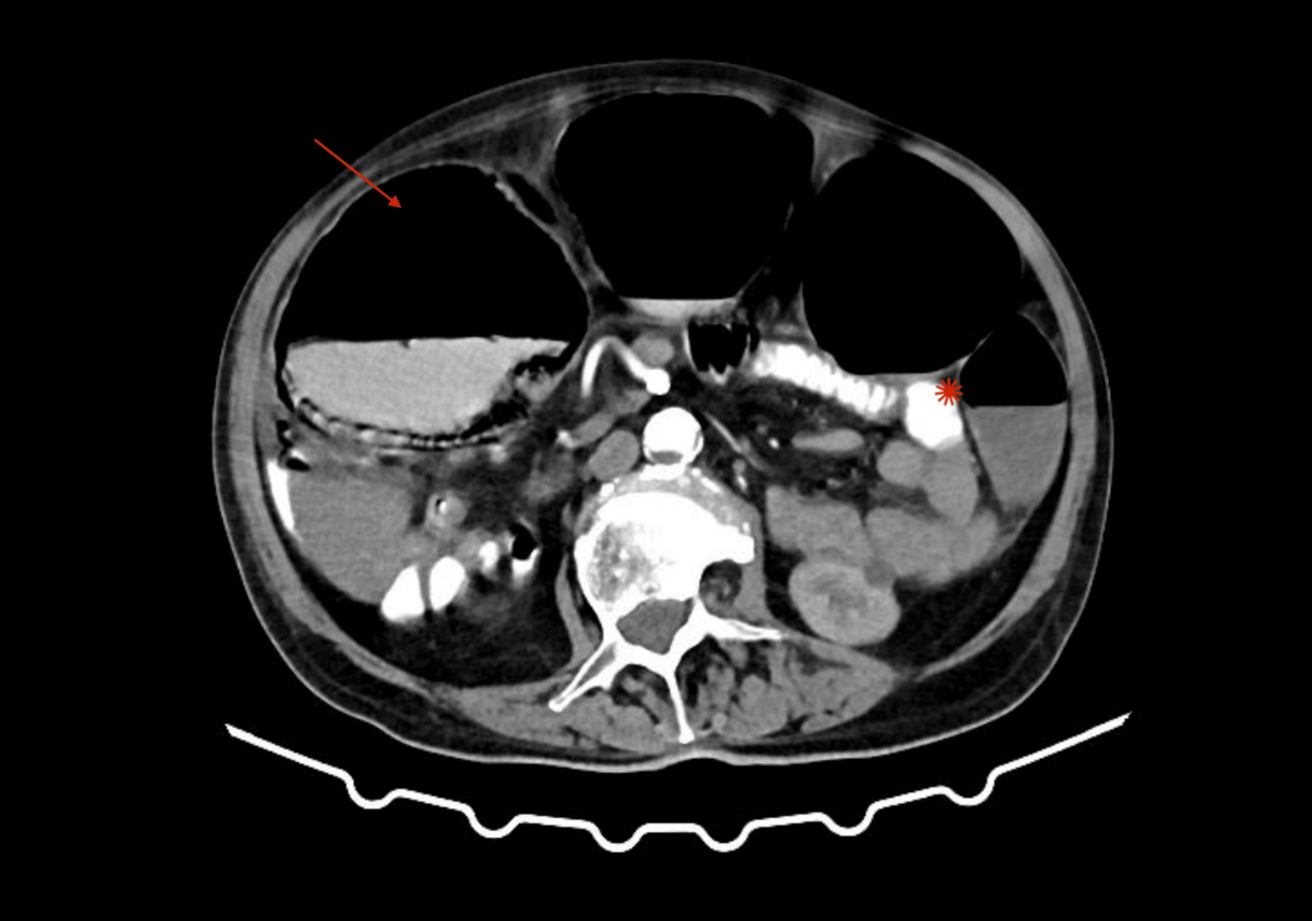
Computed tomography of the dilated large bowel (arrow), with normal small bowel (star). Large bowel obstruction with distention of the ascending and transverse colon is evident, while the small bowel is unaffected, evident of a competent ileocecal valve.
